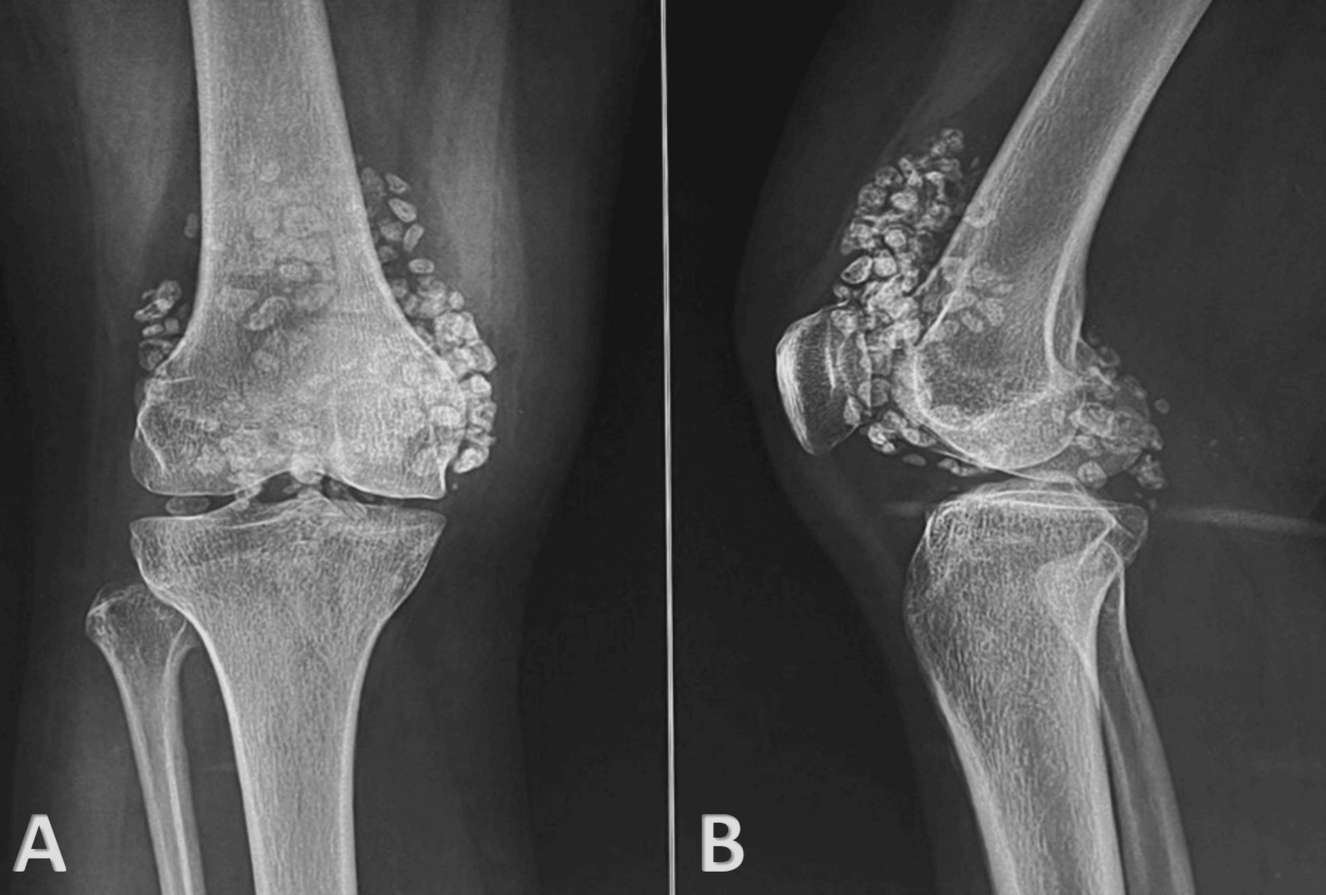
Preoperative radiographs of the left knee joint. (A) Anterior-posterior view of the left knee joint showing multiple loose bodies. (B) Lateral view of the left knee joint showing multiple loose bodies.
